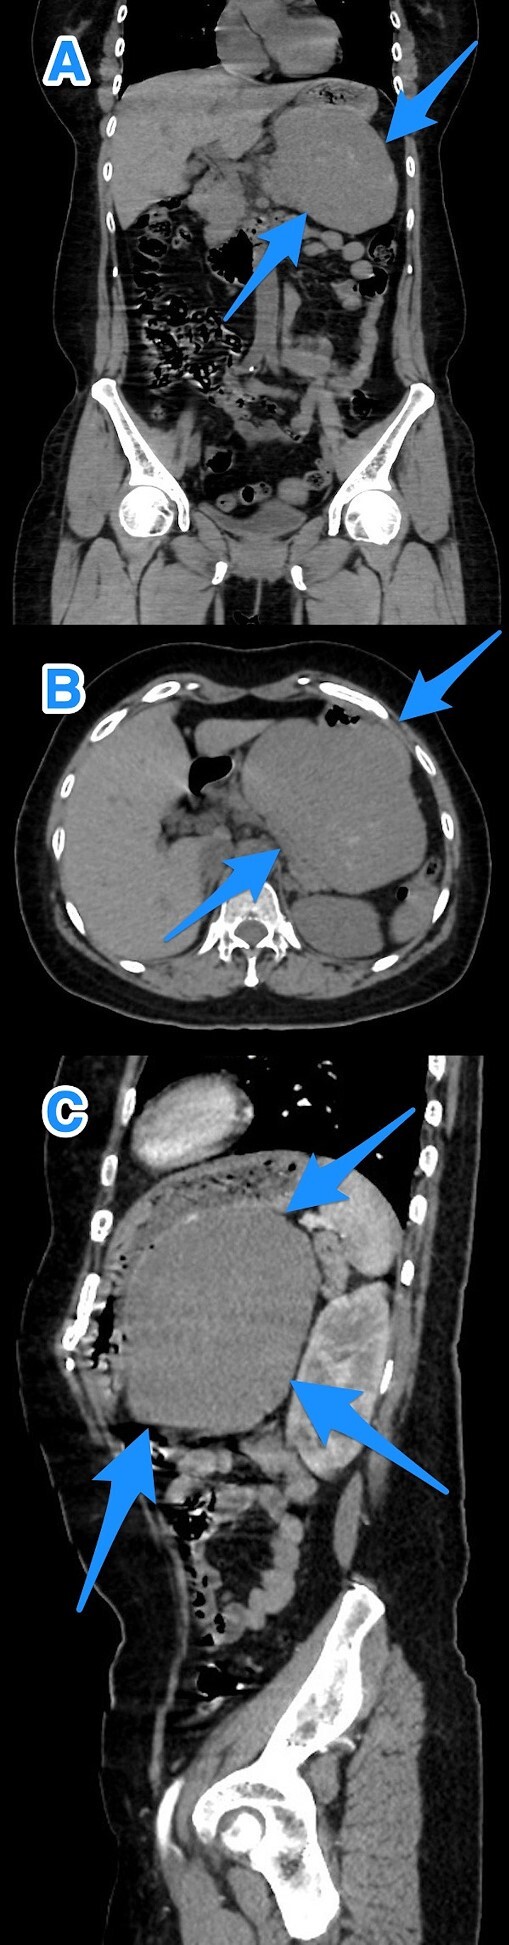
A multi-view computed tomography scan of the abdomen (A–C) showing a well-defined large gastric fundal/body submucosal heterogeneously hypoenhancing soft tissue mass lesion of the posterior gastric wall (arrows).
